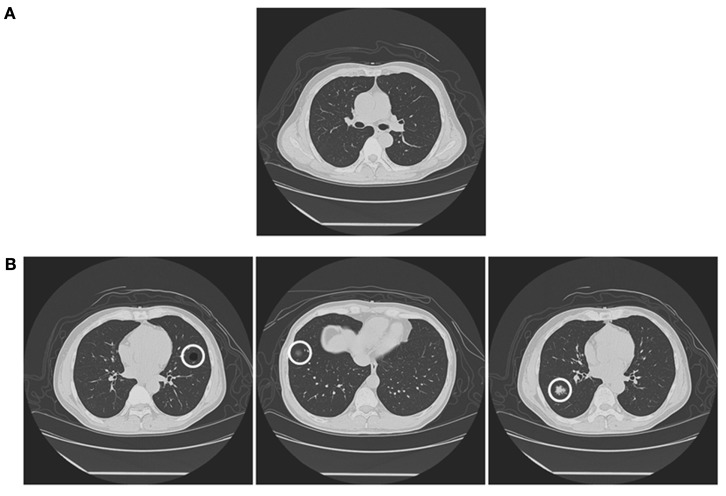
Samples of stimuli in the experiment. (A) A background CT image (target-absent image), and (B) target-present images, including a bulla-present image (Left), a GGN-present image (Center), and a cancer-present image (Right). Each target lesion is marked by a white ring in this figure. Note; the white ring was not presented in the experiment.
